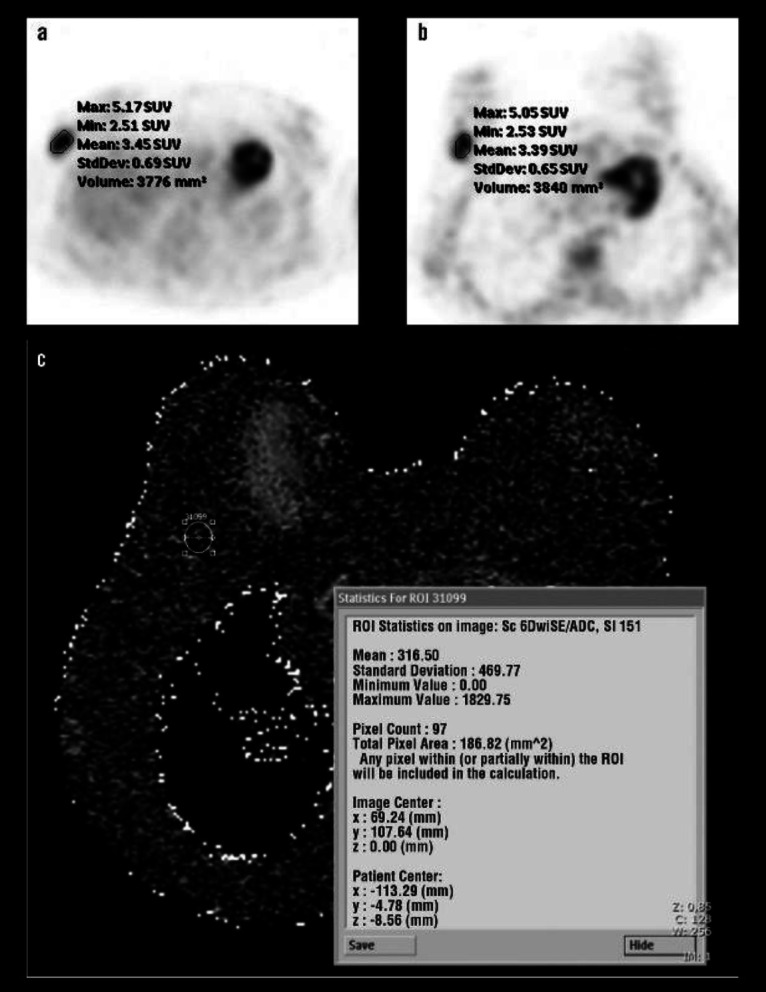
(a) Transaxial PET/CT images in supine position, (b) images at prone position obtained shortly after supine position images for accurate localization of primary tumour and (c) ADC map in diffusion-weighted imaging display, in a 40 year old woman with IDBC and 2.5 cm tumour size, grade 3, ER-PR positive, 1+ Her2/*neu* status in the right breast. ROIs are shown on (a) and (b) for measuring SUV_max_, and on (c) for measuring the mean ADC value
